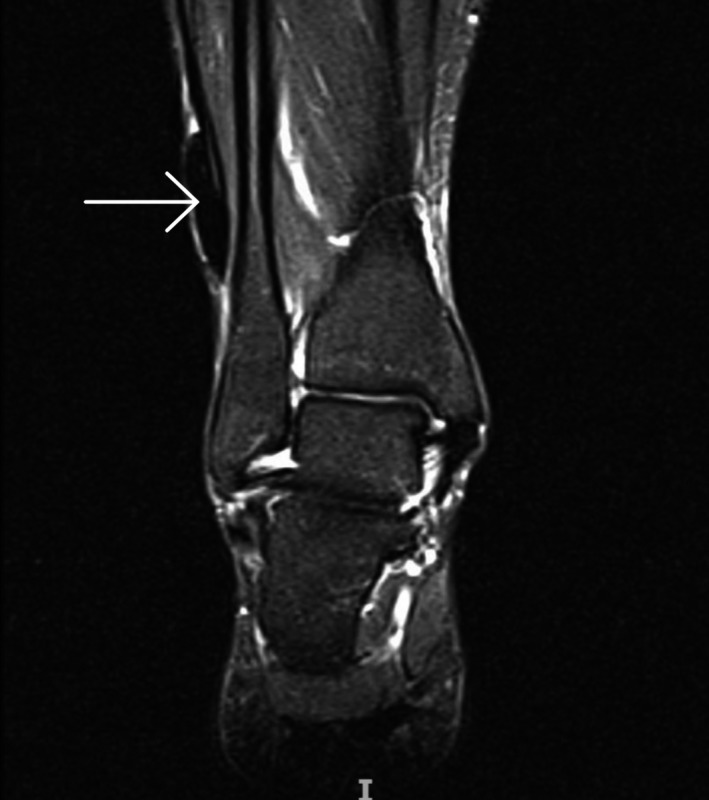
T2 sequence on the MRI showing a hypointense area of 3 × 1 cm in the medial lower extremity
